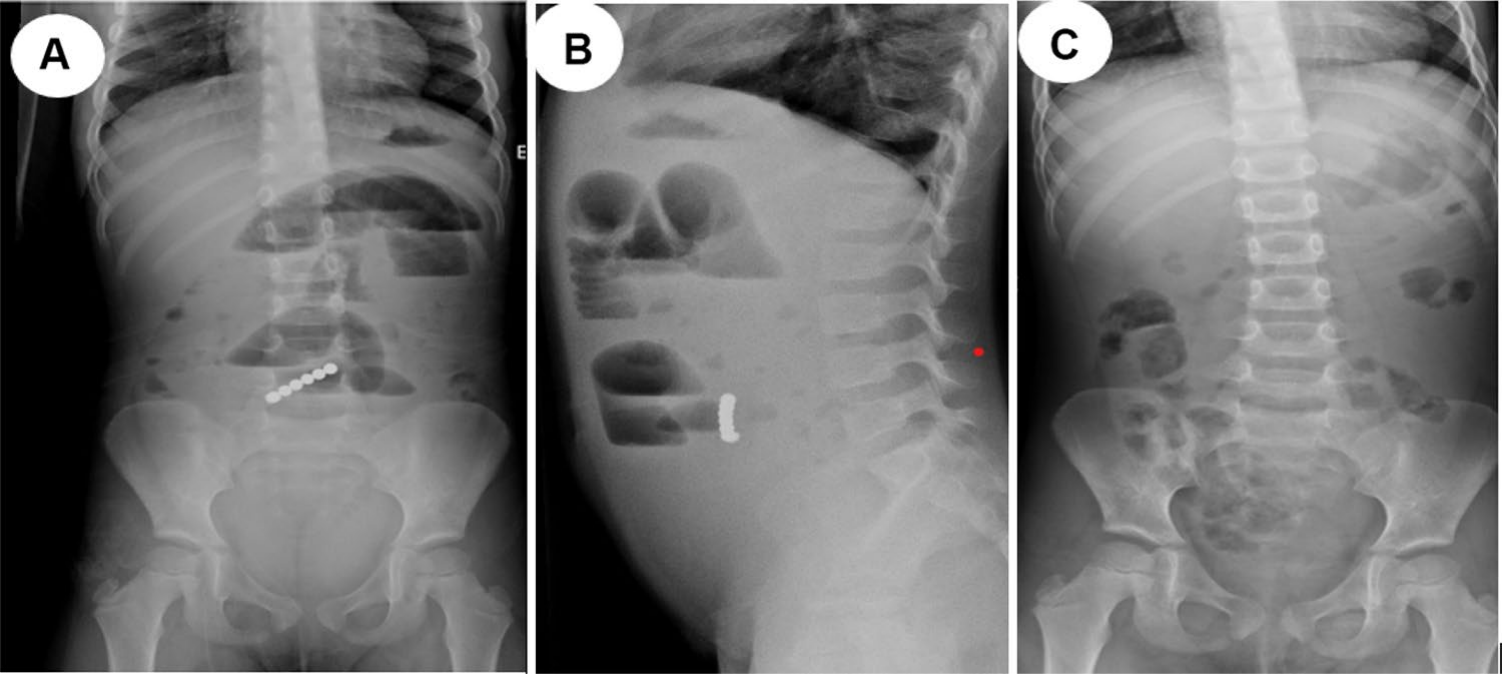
Preoperative radiograph showing multiple magnets causing intestinal obstruction (multiple air fluid levels) (A) AP view; (B) Lateral view; (C) after surgical removal of magnets.
